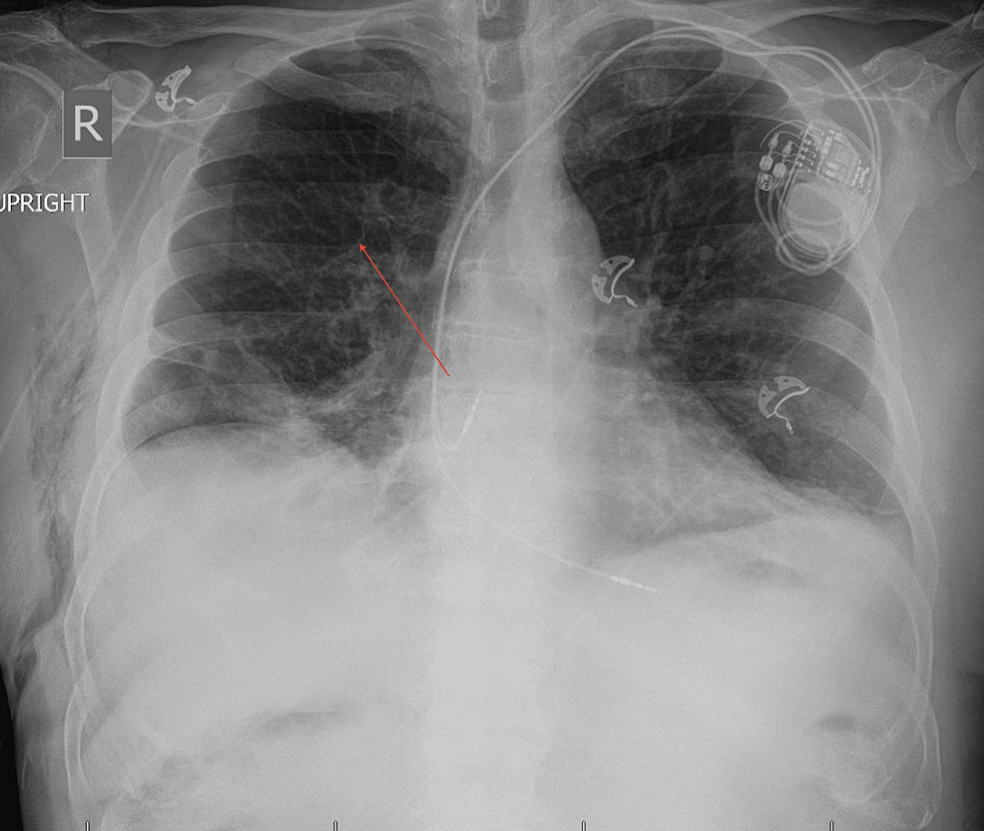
Chest X-ray demonstrating contralateral pneumothorax to the pacemaker, as indicated by the arrow
